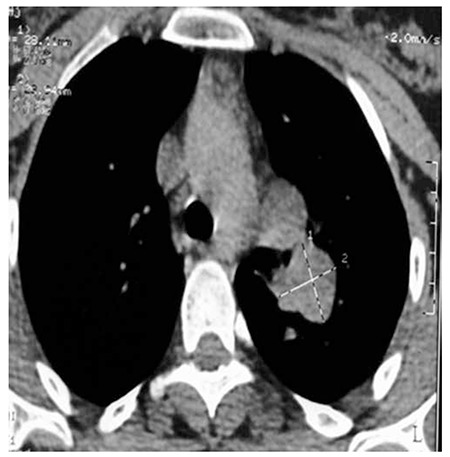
Computed tomography scan showing a large mass in the left lung field
